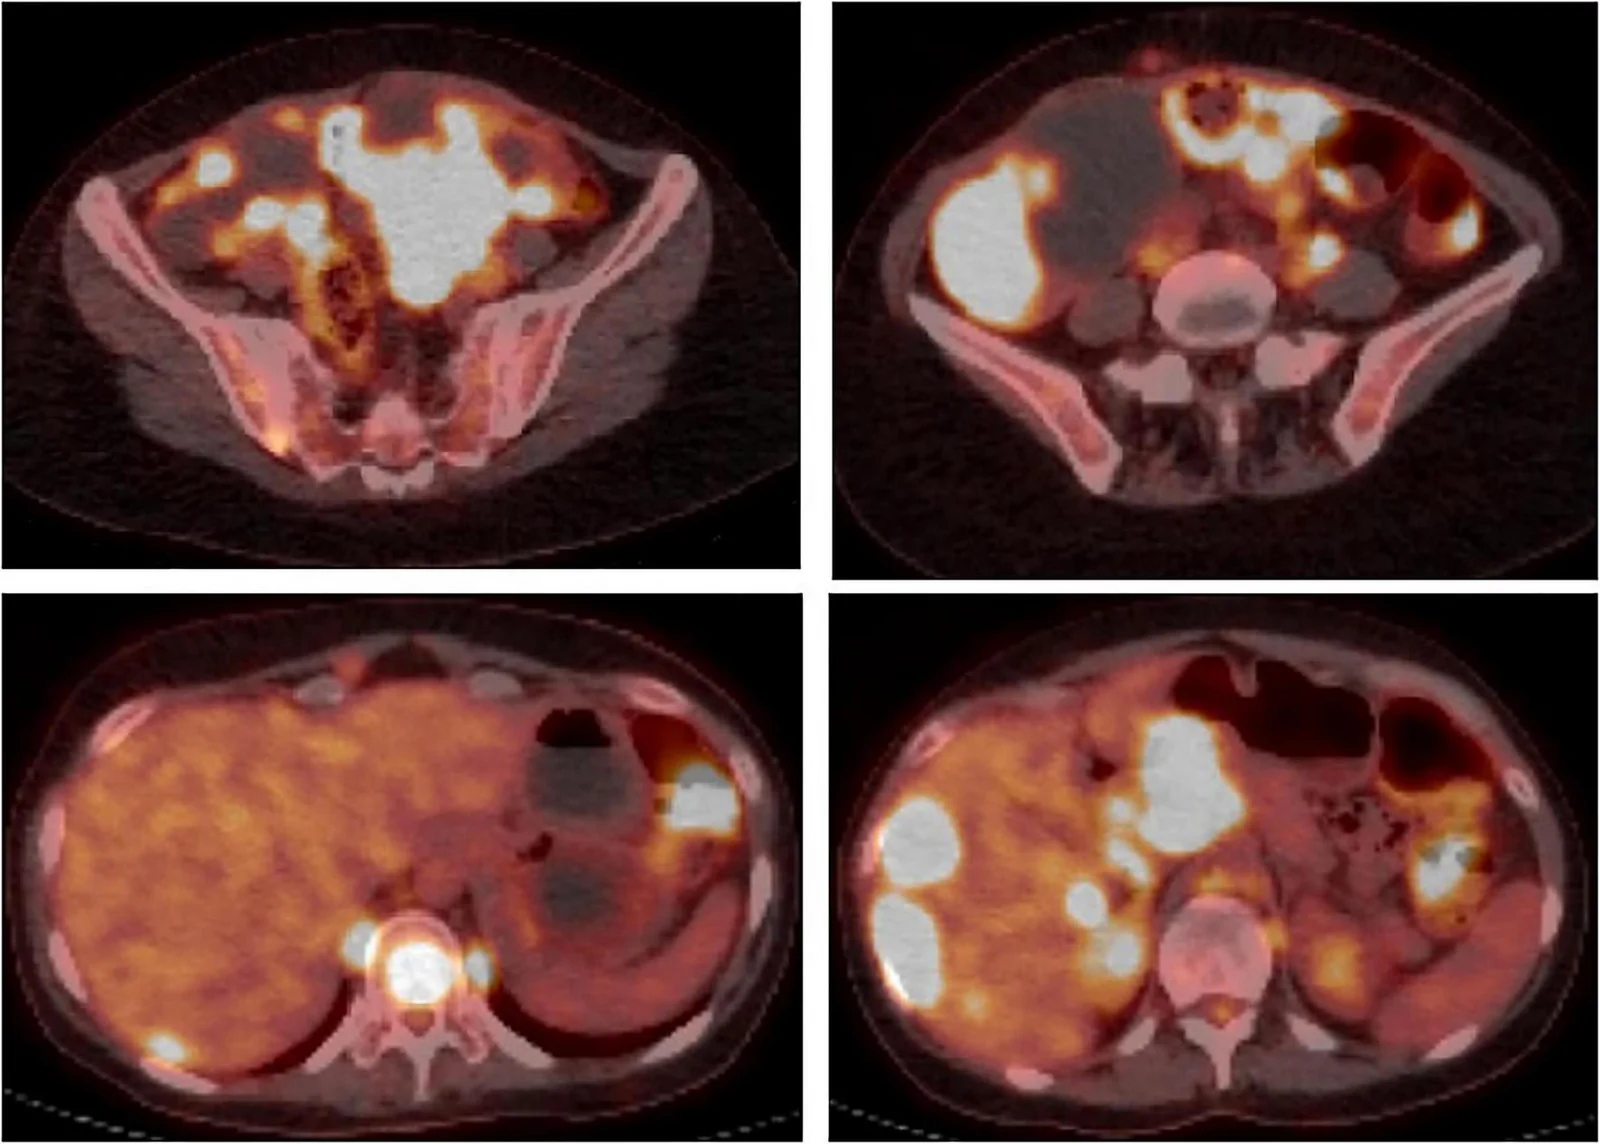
PET FDG CT scan at diagnosis.
